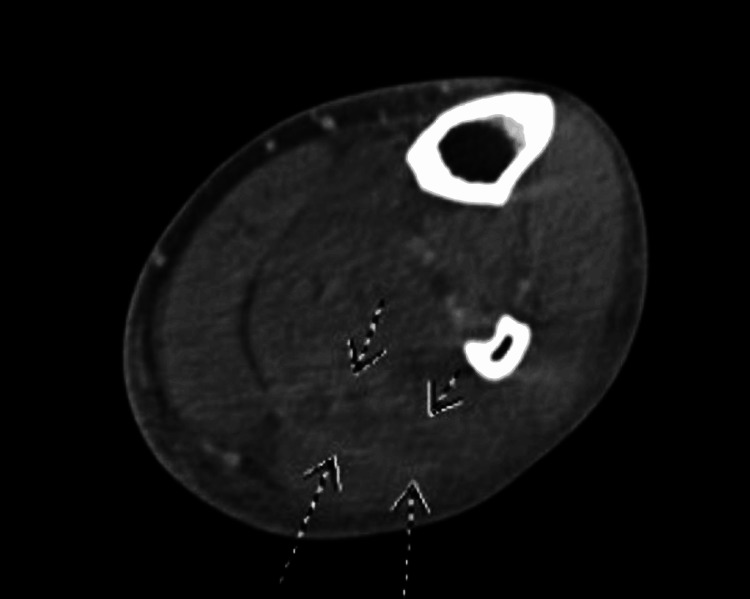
Left lower extremity CT with fluid collection visualized, transverse view.
